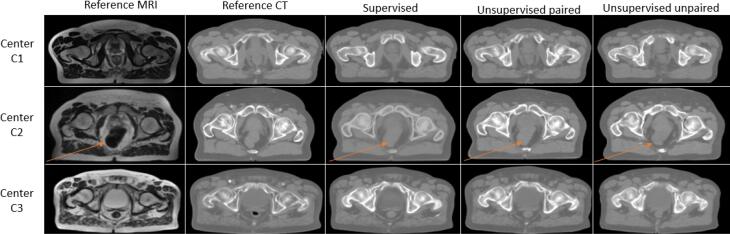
Image results with 3D cGAN according to the chosen learning process: supervised, unsupervised with paired data and unsupervised with unpaired data. The orange arrow shows the presence of gas in the rectum for MRI and the abscence of gas in the sCTs.
